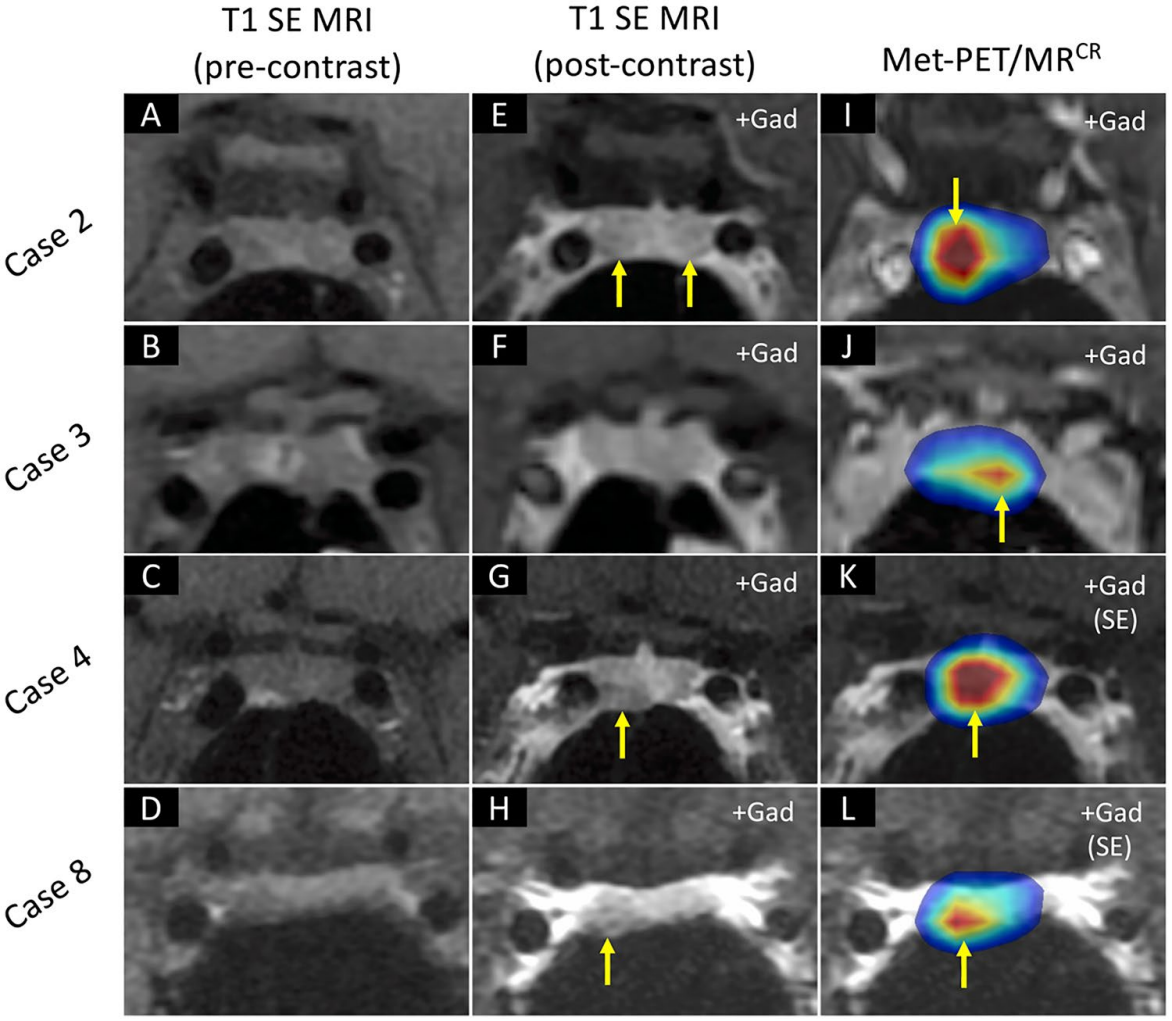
MRI and Met-PET findings in cases 2, 3, 4 and 8. A–H Pre- and post-contrast coronal T1 SE MRI show equivocal appearances in four patients, identifying either no abnormality or possible single or multiple lesions (arrows). I–L In contrast, in all four subjects Met-PET/MR^CR^ demonstrates a single focus of intense tracer uptake which was subsequently confirmed at transsphenoidal surgery to be the site of a microprolactinoma. Postoperatively, all patients remain normoprolactinemic and eupituitary. *FSPGR* fast spoiled gradient recalled echo, *Gad* gadolinium, *MRI* magnetic resonance imaging,* Met-PET/MR*^*CR*^
